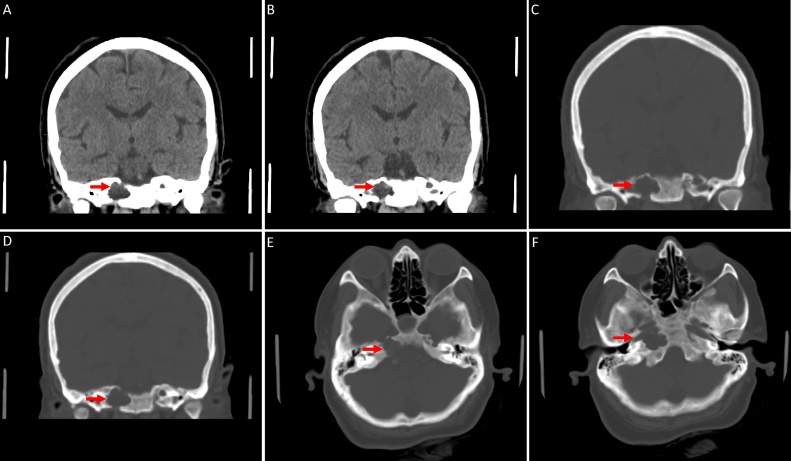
Nonenhanced CT brain; (A-B) Coronal brain window. (C-F) Coronal and axial bone window. (A-F) There are bilateral, well-defined, lytic, expansile, fluid-containing lesions in the petrous apices with bone dehiscence of the inner cortex of the petrous bone.
